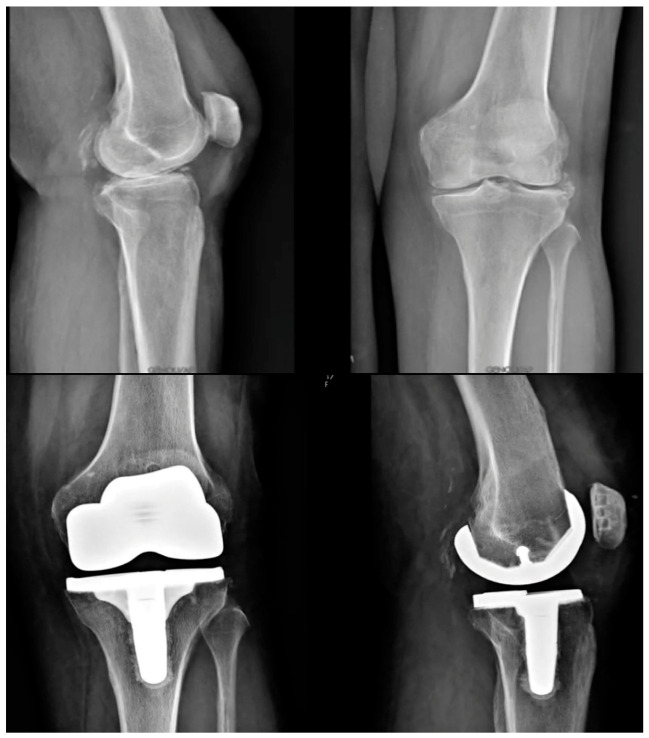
Preoperative (top) and postoperative (bottom) X-ray images of a patient undergoing individualized TKA with the Origin^®^ system.
